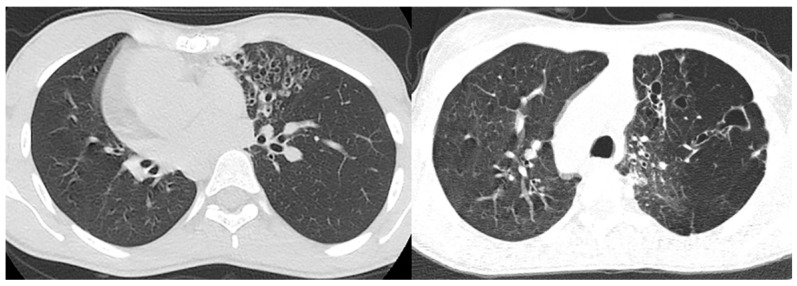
High-resolution CT of the lungs in two patients with confirmed Kartagener syndrome reveal the characteristic bronchiectasis (signet ring appearance) predominantly in the left lung.
